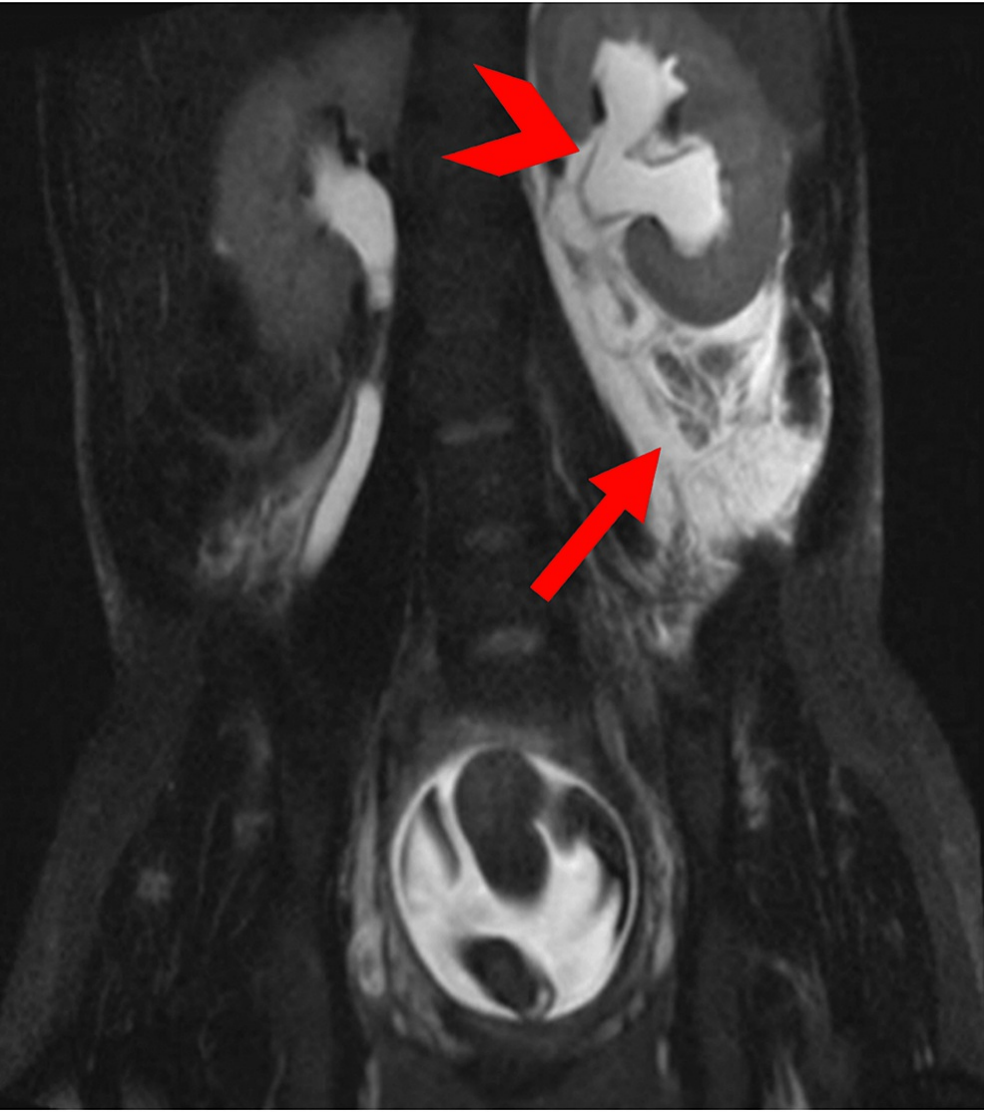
Abdominopelvic MRI (coronal view) The image shows the left hydroureter (arrowhead) and fluid extravasation surrounding the left kidney (arrow).
